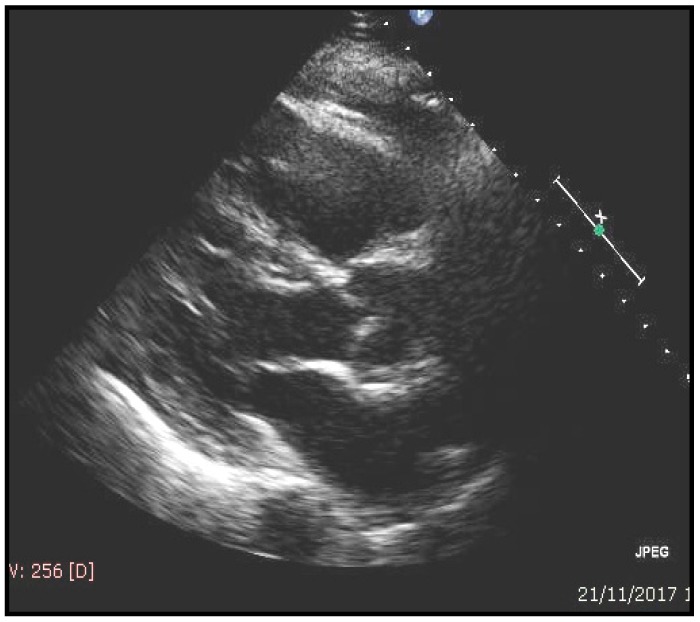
A typical example of sarcopenia and echocardiographic evaluation in an 82-year-old male patient. A reduction of strength and mass muscle is associated to “non-functional” cardiac hypertrophy (LV = left ventricular; *E/e*^1^ = echocardiographic transmitral early peak velocity (*E*) by pulsed wave Doppler over *e*^1^ (*E/e*^1^) represent a noninvasive surrogate for LV diastolic pressures for grading a diastolic dysfunction).
